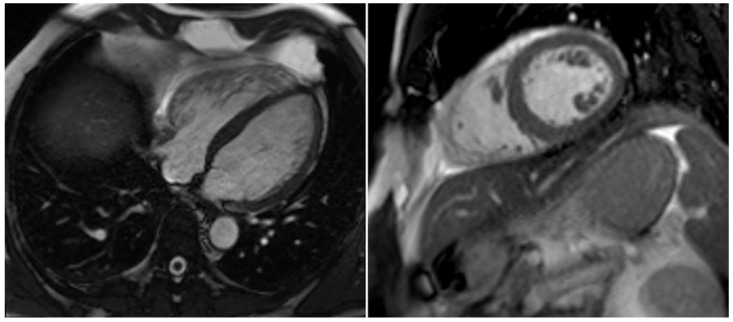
Four chamber view (left side) and short axis view (right side) of a cardiac magnetic resonance imaging (MRI) four weeks after the procedure. Right ventricular failure could be excluded; however, prominent trabeculation was present.
